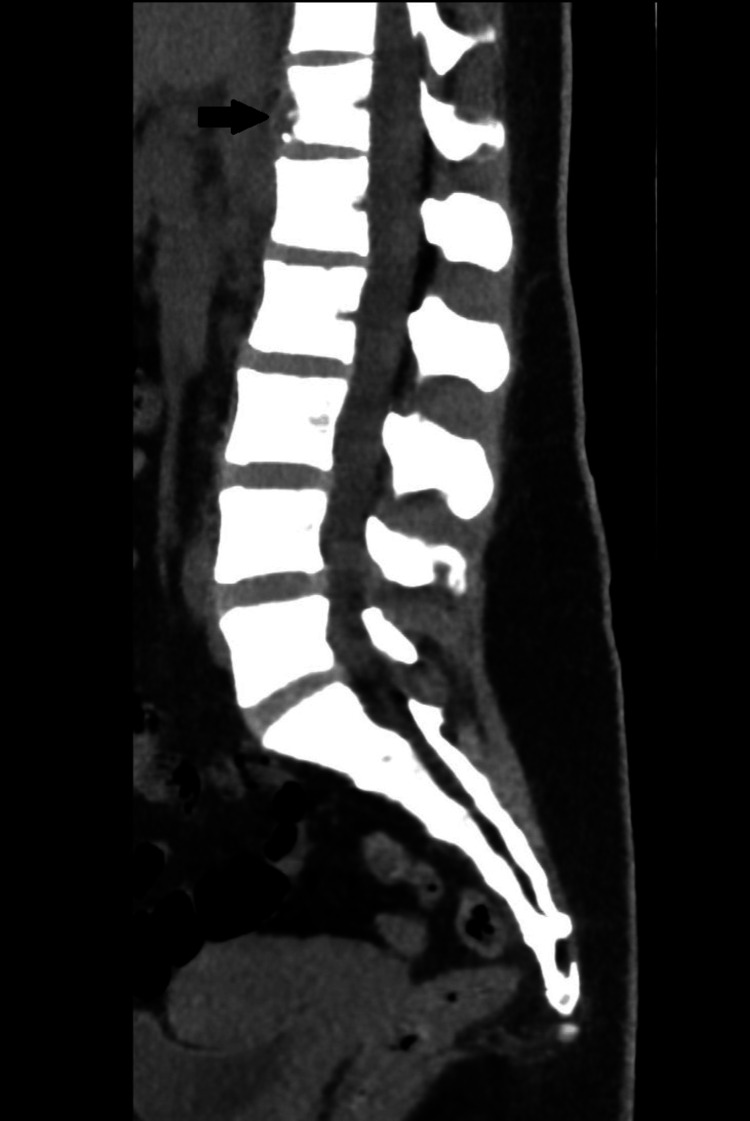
Lumbosacral computed tomography: sagittal plane Radiolucent lesion involving the T12 vertebral body (black arrow).
